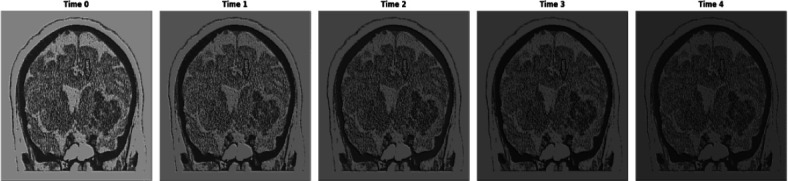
Findings: Serial coronal CT images demonstrate a progressive resolution of a previously identified right basal ganglia hemorrhage. At Time 0, a well-circumscribed hyperdense lesion with surrounding edema and mild mass effect is visualized. As the timeline progresses from Time 1 to Time 4, there is a steady decrease in hematoma density and volume, with significant resolution of mass effect and peri hematom al edema. The ventricular anatomy becomes more defined, and midline structures return to near-normal alignment. No new hemorrhagic events, hydrocephalus, or ischemic changes are observed. The imaging appearance at Time 4 suggests the hematoma has entered a chronic phase, characterized by hypodensity and near-complete resorption. Impression: Progressive resolution of right basal ganglia intracerebral hemorrhage over the observed interval. No evidence of rebleeding or secondary complications. Current findings are consistent with late subacute to chronic hemorrhage stage. Recommendation: Continue clinical monitoring. Optional MRI may be considered to assess gliosis or underlying structural changes, if clinically warranted.
